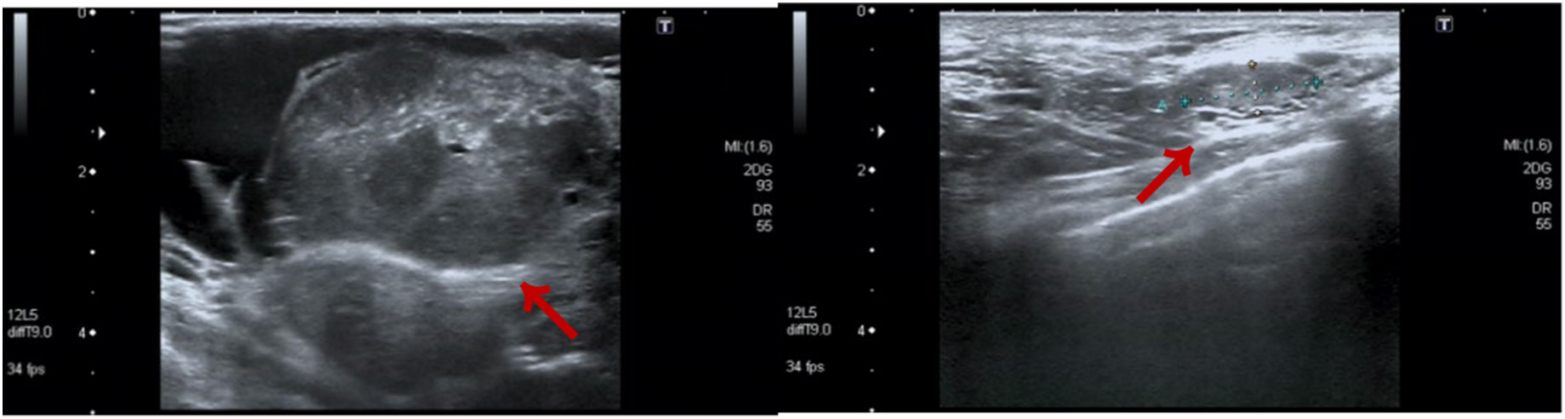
Color ultrasonography evaluation of the patient before surgery.
